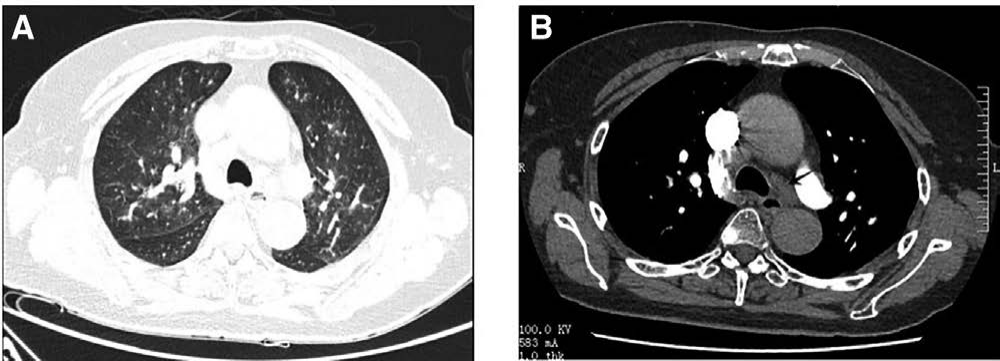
Chest computed tomography of the patient with pulmonary tumor microangiopathy as the disease aggravated. (A) The lung window showed that thickened lung texture and multiple patchy shadows. (B) The window of mediastinum revealed that multiple lymph nodes were enlarged.
